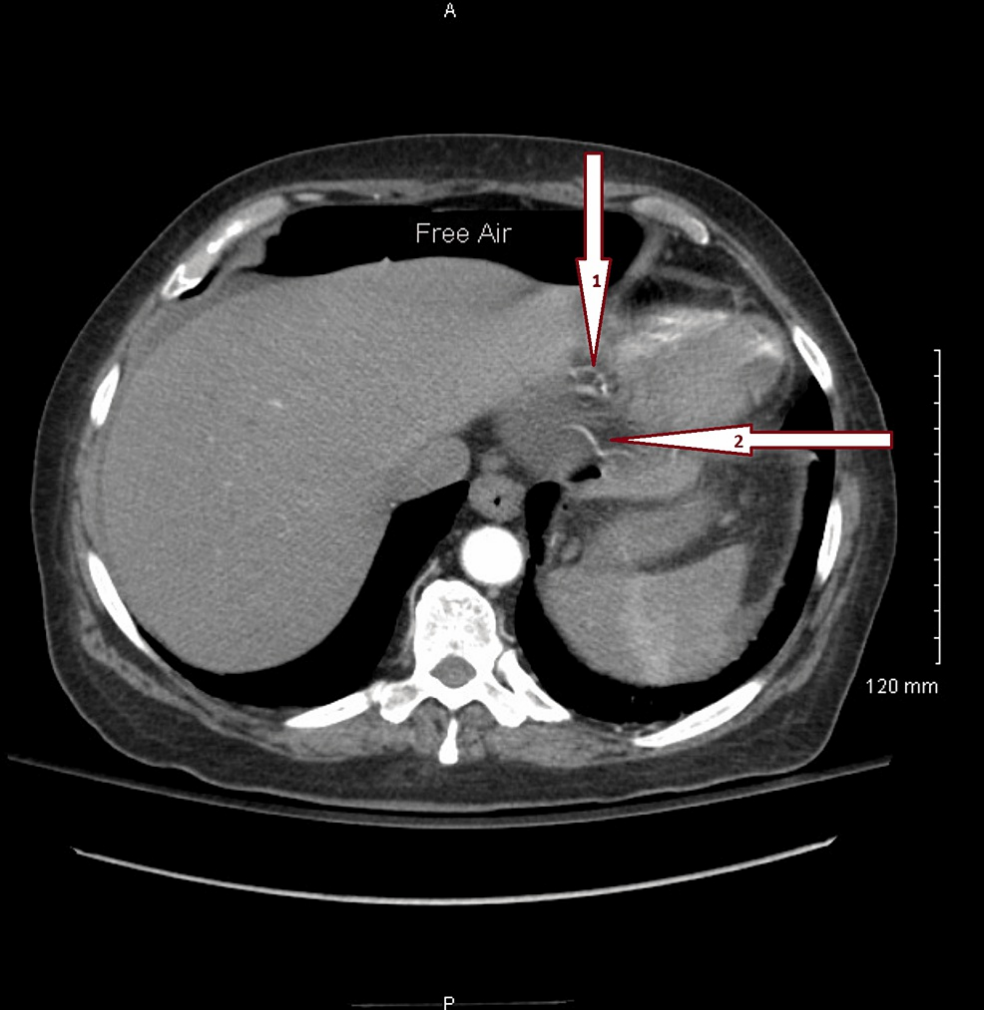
Abdominal CT where possible ulceration occurred (pylorus) Important to note that the black shade above the liver is free air. The free air was an indication that there was a perforation. Arrow 1 from the top is the area where said perforation occurred. Arrow 2 points to where possible perforation might have occurred.
